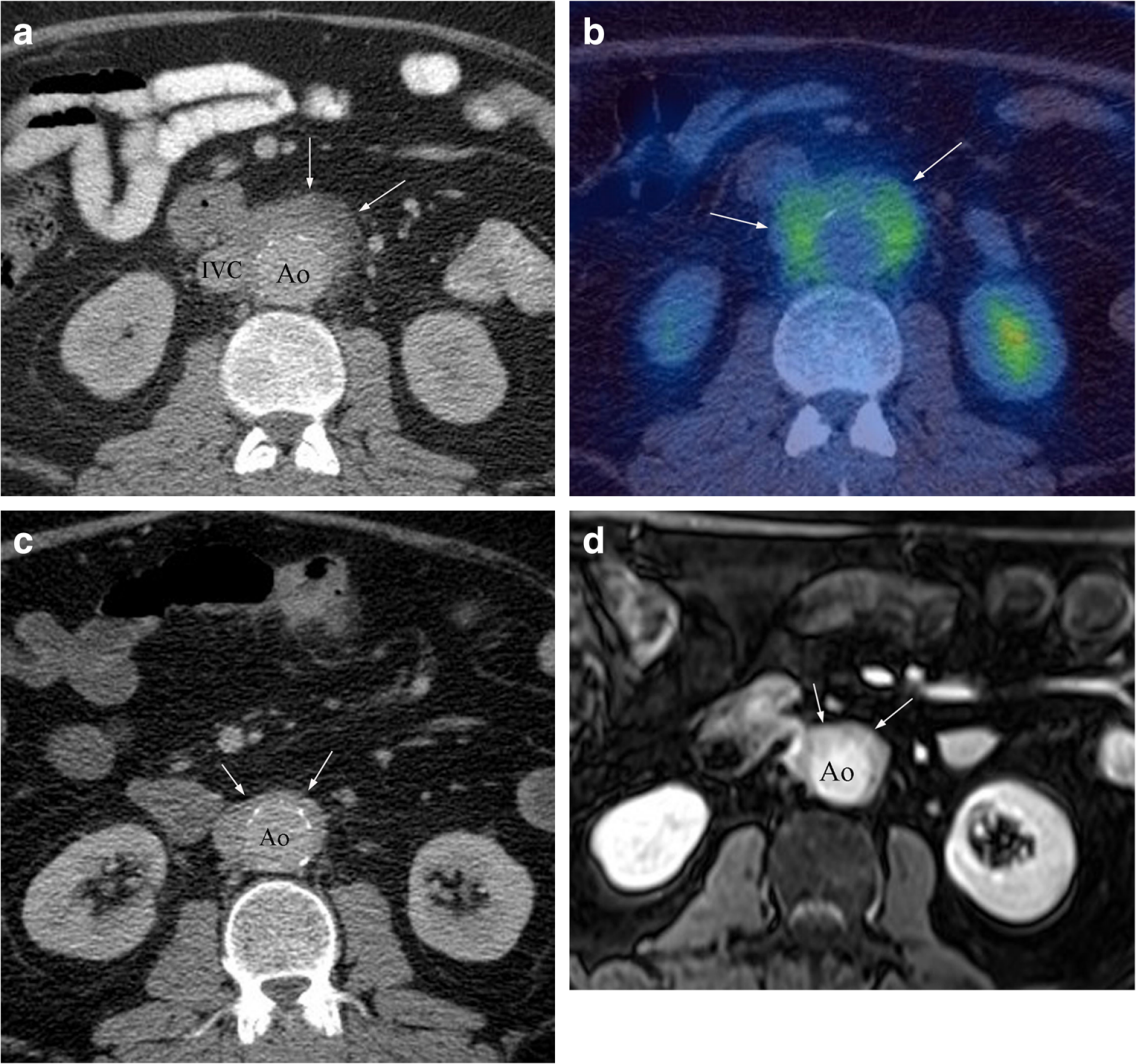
Example of IgG4 retroperitoneal fibrosis. a Axial CECT showing enhancing soft tissue partly encasing the IVC and aorta (*arrows*). b Axial FDG PET/CT showing increased FDG uptake in the abnormal retroperitoneal soft tissue (*arrows*). c Axial CECT in the same patient, with reduced periaortic soft tissue, following treatment with corticosteroids. d Corresponding T1FS post-contrast MRI showing residual enhancing soft tissue around the aorta following steroid treatment (*arrows*)
